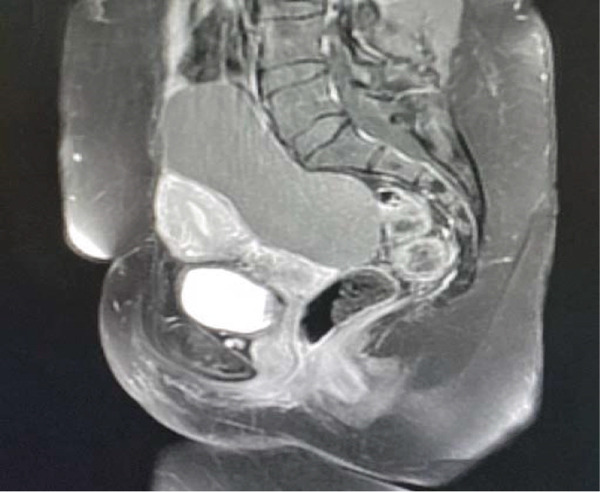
MRI postcontrast showed multilocular appearances with a few smooth septations, which showed postcontrast thin enhancement.
